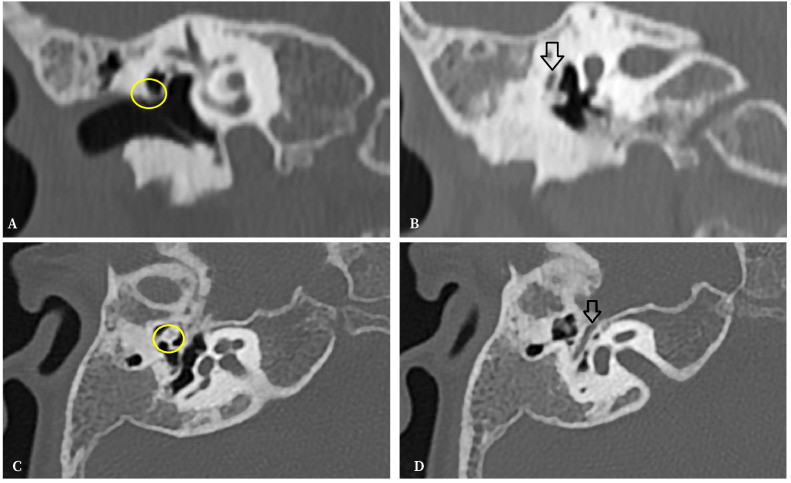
Computed tomography. A. Coronal slice. Hypoplasia of the tympanic cavity and the defect in ossicular chain with the incus attached to the tympanic wall (circle). B. Coronal slice. Abnormal course of the facial nerve (arrow). C. Axial slice. Hypoplasia of the tympanic cavity and the ossicular chain with fixation of incus to the tympanic wall (circle), and lack of mastoid pneumatisation. D. Axial slice. Abnormal course of facial nerve (arrow).
